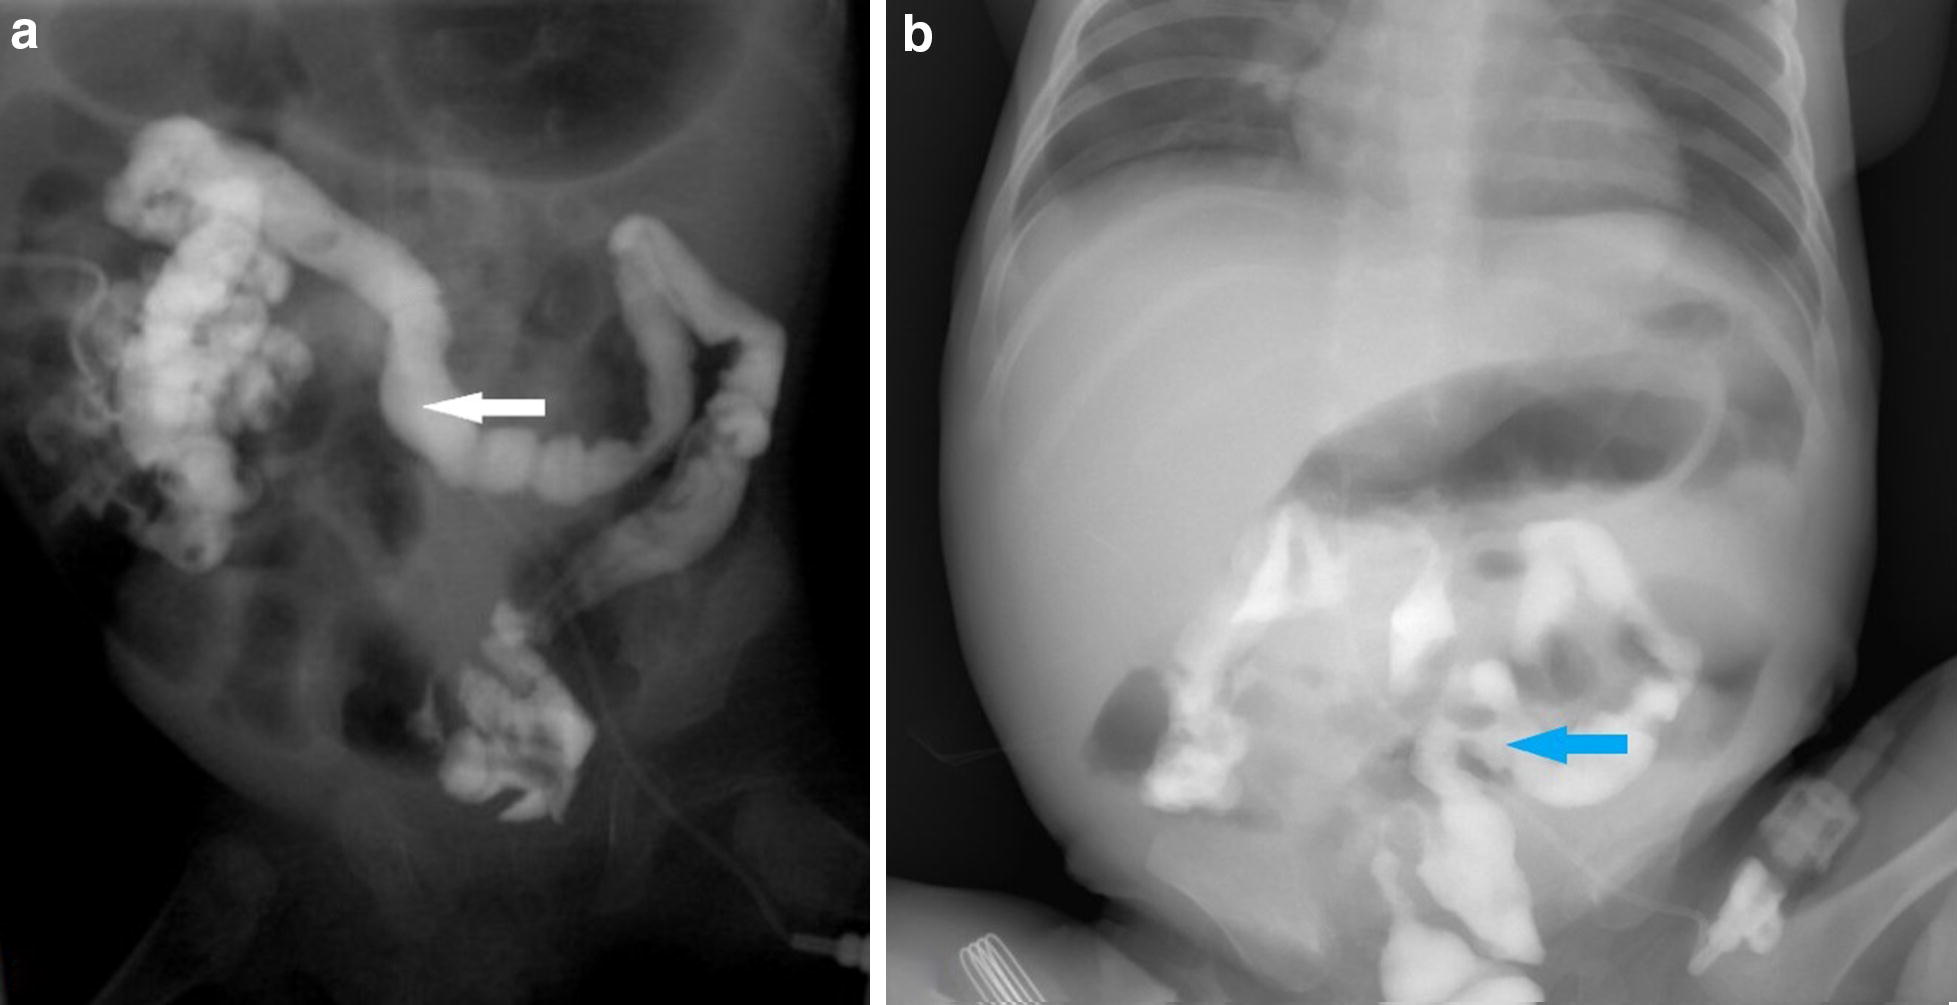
a The distal stoma imaging. The maximum width of the transverse colon was approximately 0.8 cm (the white arrow). b The upright plain abdominal radiograph. The image was taken 24 h after the previous imaging. The contrast medium was stranded in the colon (the blue arrow)
